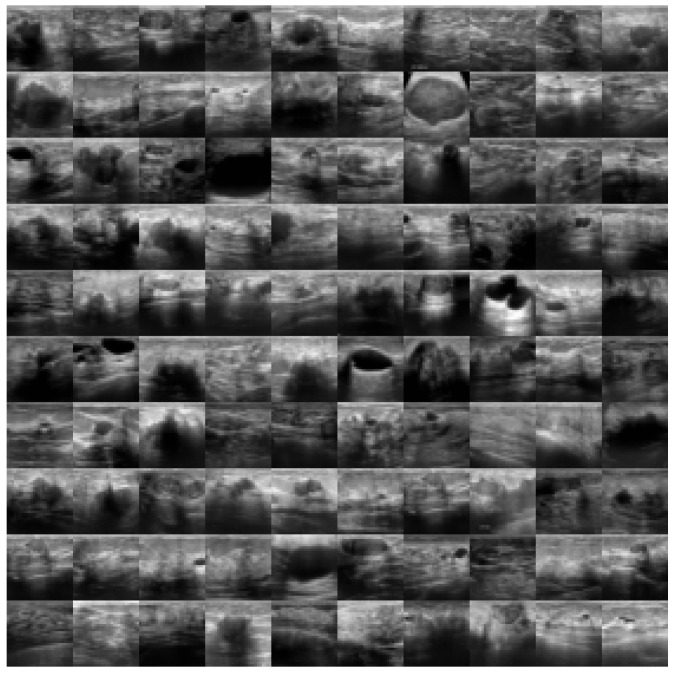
A 10 × 10 montage of samples from ultrasound breast images extracted from the training set, encompassing three distinct classes: normal, benign, and malignant, from the BreastMNIST dataset.
